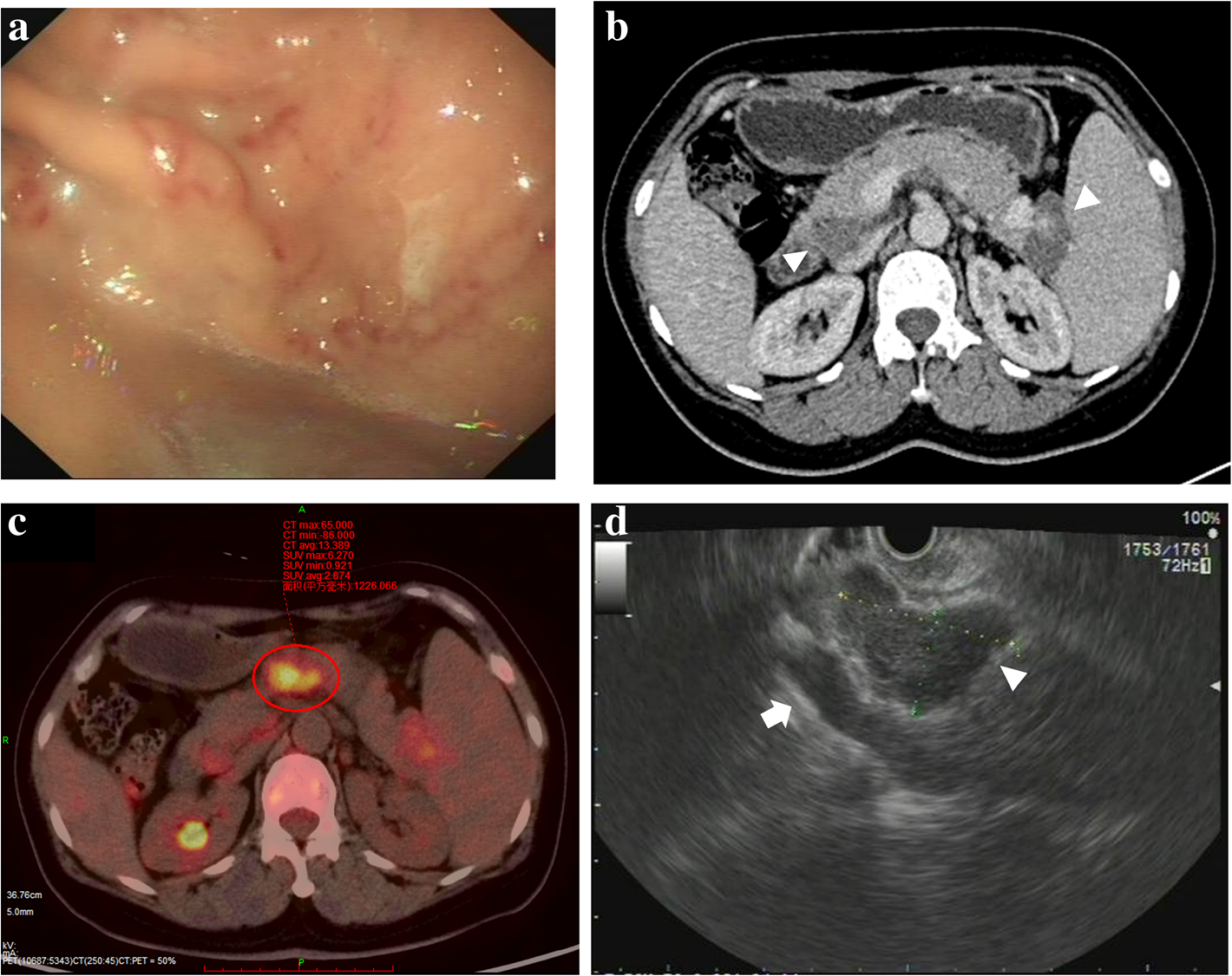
(a) Gastroduodenoscopic images show gastric fundal varices with characteristic red color signs. (b) CECT scan shows masses behind the head, body, and the tail of the pancreas (*arrow heads*). (c) PET/CT scan shows hypermetabolic lymphadenopathy in the retropancreatic area and at the splenic hilum (size 42.8 mm × 21.2 mm). (d) EUS shows a hypoechoic mass at the splenic hilum (*arrow head*) compressing the splenic vein (*arrow*)
